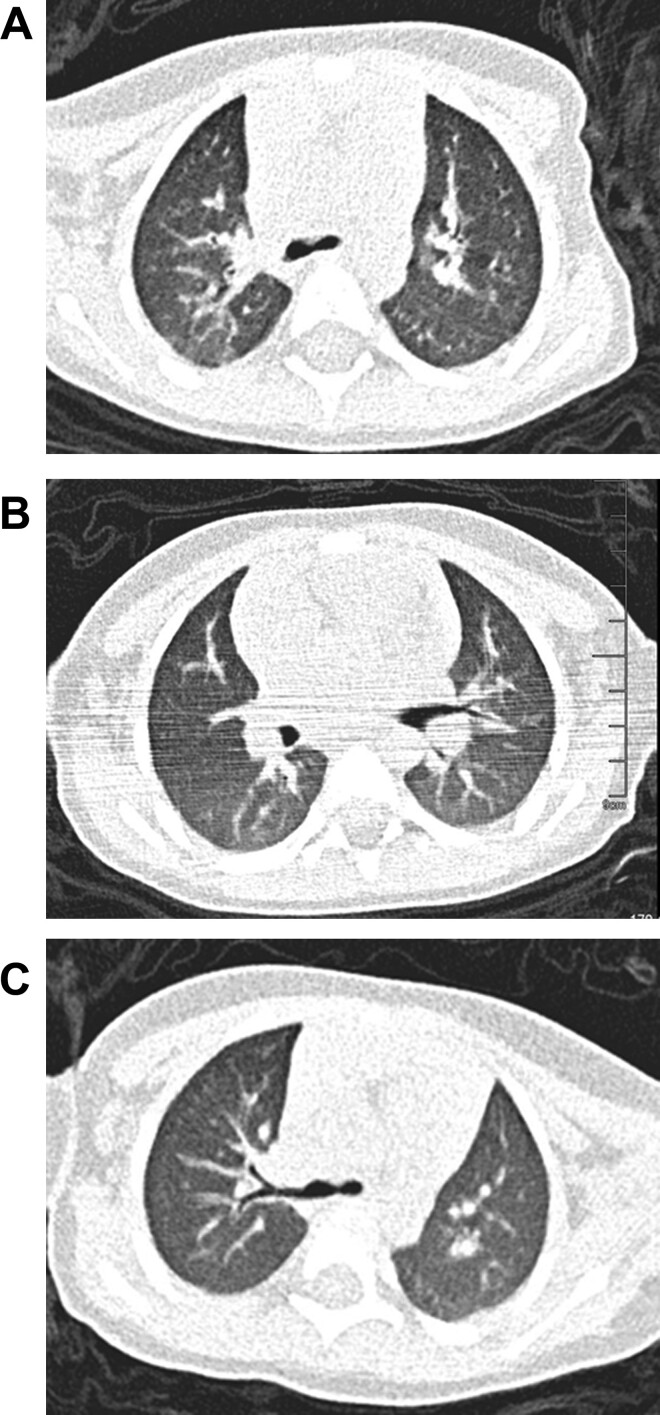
Chest CT images of the child with COVID-19. (A) Multiple inflammations in both lungs; large areas of GGOs in both lungs, possibly caused by poor breath-hold performance (day 1 of hospitalization). (B) Less inflammation in the right upper lobe than that on day 1 (day 8 of hospitalization). (C) Chest CT images displayed normal lungs (day 17 of hospitalization).
